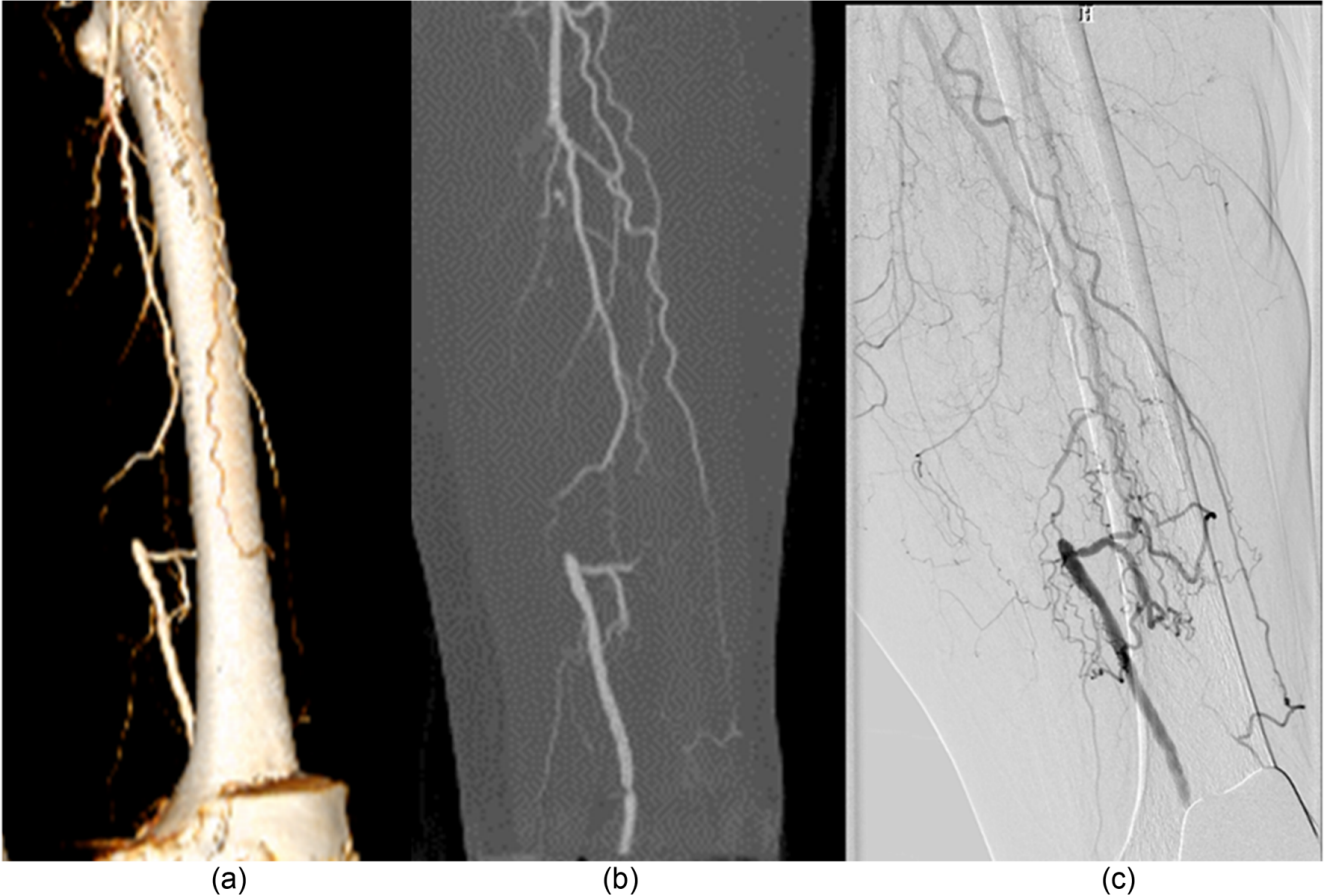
A 70-year-old female with left gangrenous toe for 3 weeks. There was an occlusion in the left proximal superficial femoral artery. The collateral artery in the distal patent superficial femoral artery was found. Panel a shows the volume rendering CTA image, panel b shows the maximum intensity projection CTA image, and panel c shows the DSA image
